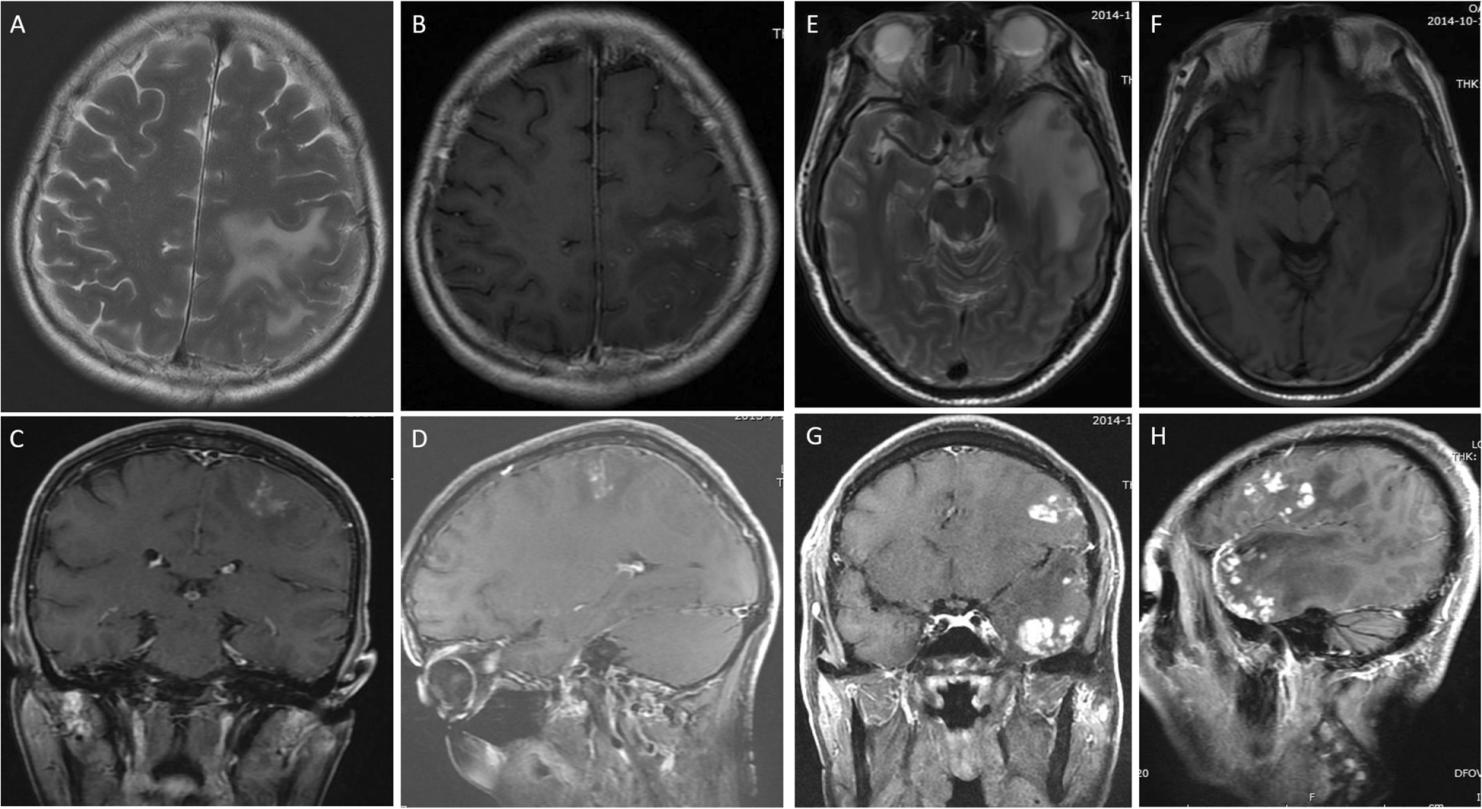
MRI features of the representative encephalic Schistosomiasis japonicum cases (a-d from Case 2, e-h from Case 4). a: T2 weighted (W) image showed a single-nodular lesion located in the left parietal lobe with perilesional edema by axial scanning. b-d: T1 W image after gadolinium injection showed single-nodular enhancement with perilesional edema by axial (b), coronal (c), and sagittal scanning (d). e: T2 W image showed edema of left temporal lobe by axial scanning. f: T1 W image showed the involvement of the left temporal lobe in multi-nodular lesions by axial scanning. g, h: T1 W image showed multiple-nodular schistosomal lesions located in frontal, parietal and temporal lobes with enhancement of the nodules
